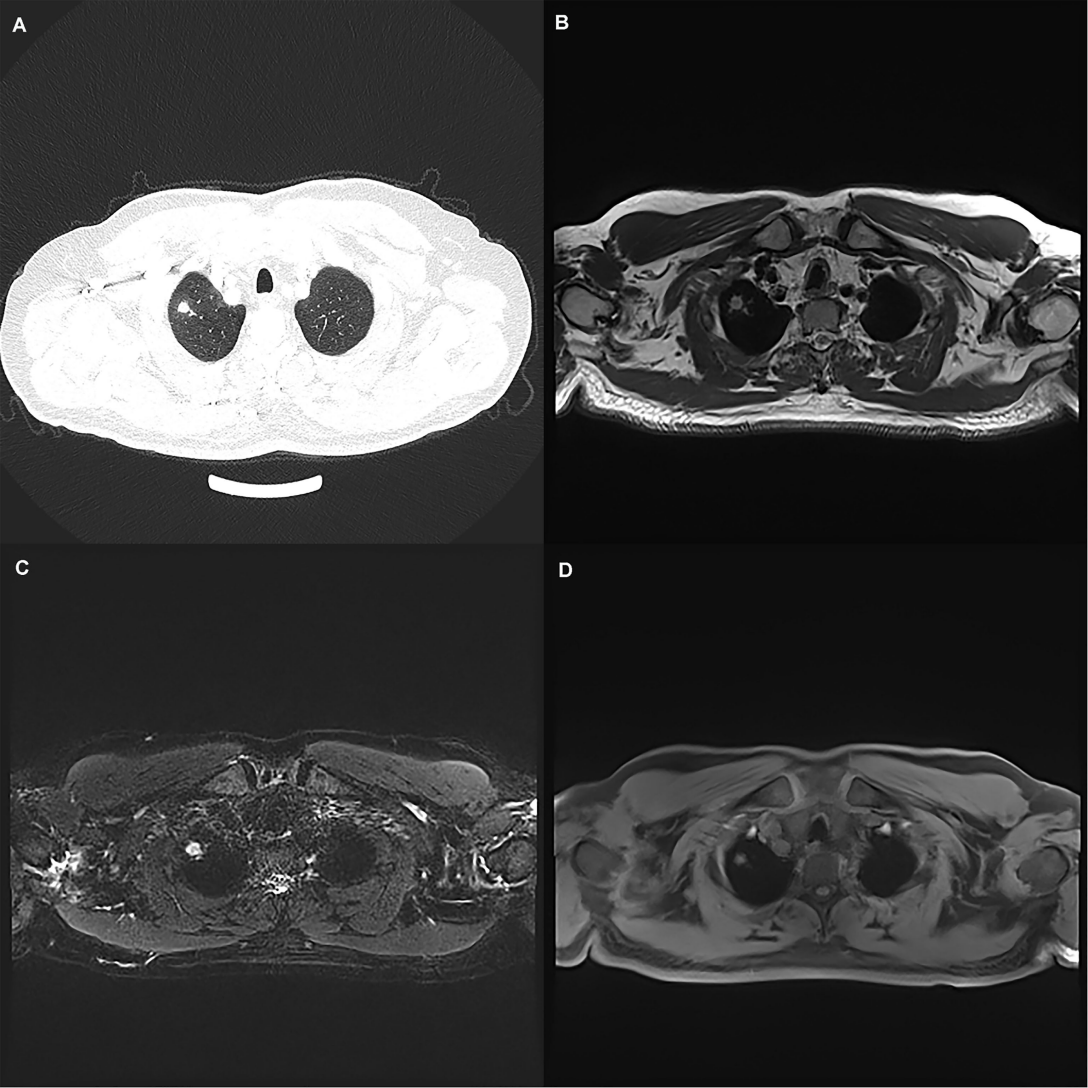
A 50-year-old man with a 7 mm pulmonary solid nodule (arrow) in the right upper lobe. The nodule is clearly seen on the computed tomography image (A) and the images from magnetic resonance image sequences T2-BLADE (B), T2-HASTE (C), and T1-Star-VIBE (D). The arrows represent the location of the nodule.
